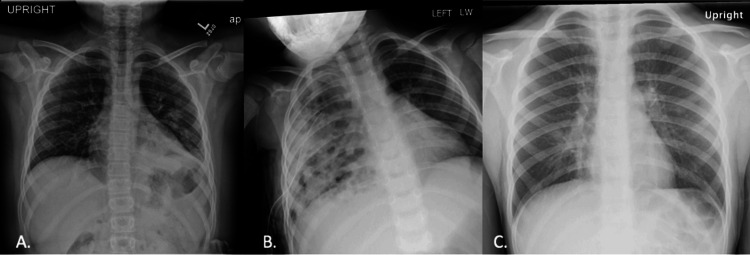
Pediatric chest radiographs A. Pediatric chest radiograph of left lower lobe pneumonia on the pre-test with patchy airspace opacities in the left lower lobe with silhouetting of the left hemidiaphragm. B. Right lung cystic/necrotizing pneumonia on the post-test with heterogeneous patchy airspace opacities throughout the right lung and a right-sided thoracostomy tube present. C. Interstitial/viral pneumonia on the six-month follow-up post-test with central predominant interstitial opacities. All images were acquired from the internal institutional Picture Archiving and Communication System (PACS).
